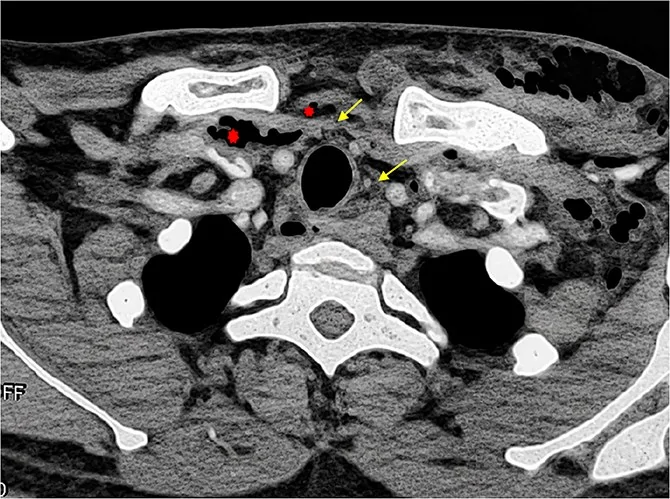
Axial contrast-enhanced thoracic CT images showing retrosternal mediastinal and left paratracheal collections (yellow arrows) associated with emphysematous infiltration of the mediastinal fat (red asterisks), consistent with descending necrotizing mediastinitis of cervical origin.
